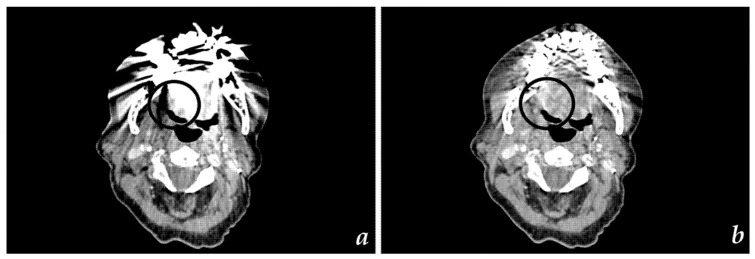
Axial CT images of (a) recurrent HNSCC of tongue edge (highlighted) without SEMAR reconstruction and (b) recurrent HNSCC of tongue edge (highlighted) with SEMAR reconstruction.
